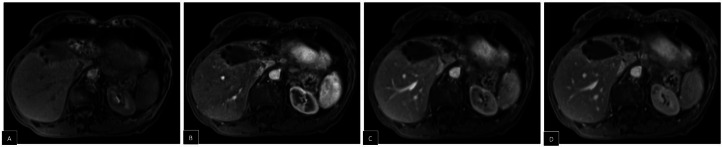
Post-operative hepatic MRI. T1-weighted precontrast image (A) and post-contrast images in arterial (B), portal (C) and delayed (D) phases reveal an absence of invasive or pathologic contrast enhancement suggesting no residual tumor.
